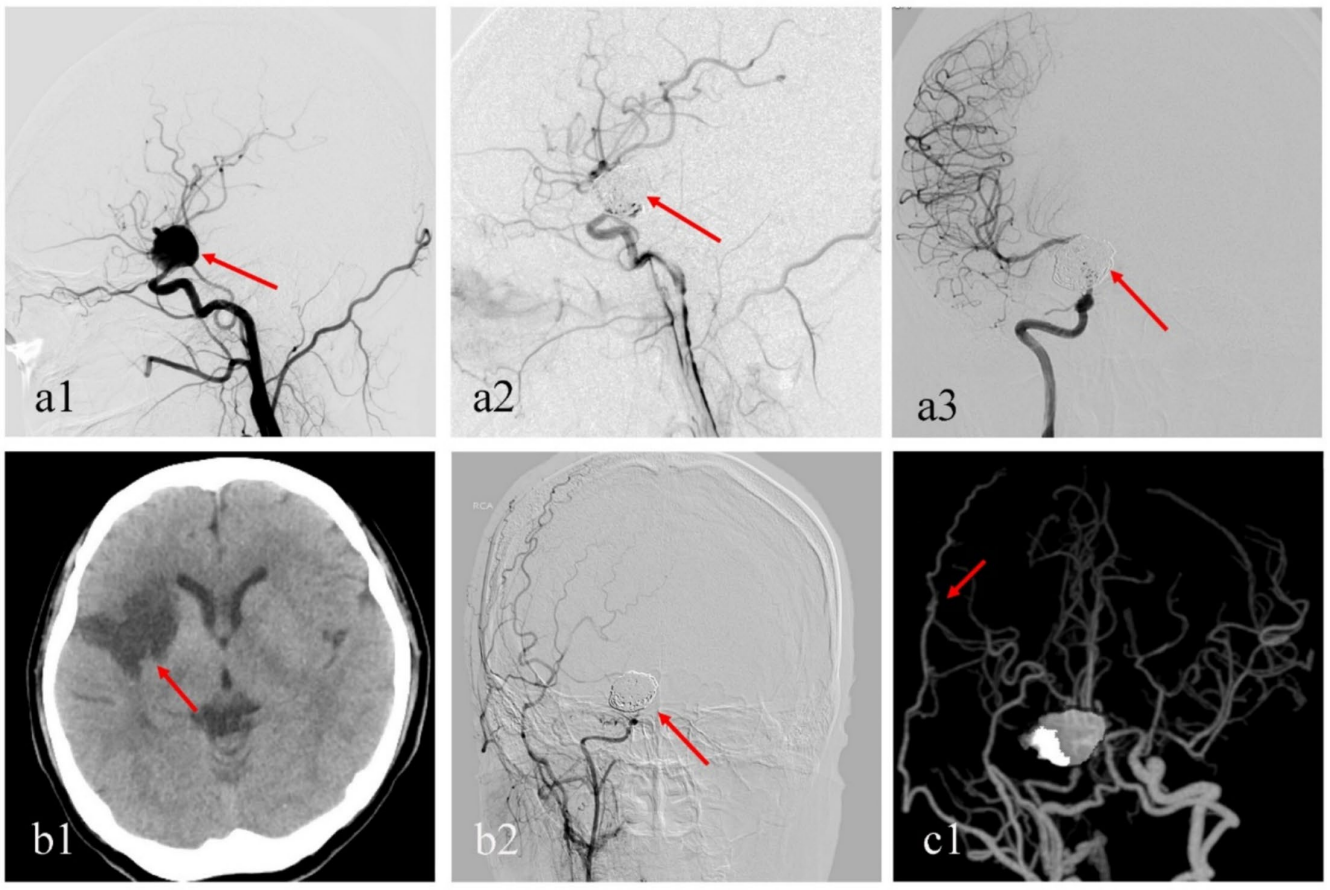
A female patient with a giant aneurysm at the distal end of the right internal carotid artery, approximately 25 mm in size (a1). She underwent a PED (3.0*35 PED Classic) combined with coiling, and immediate postoperative angiography showed aneurysm occlusion (a2). At the 3-month follow-up, the aneurysm was complete occlusion, but there was a suspicion of parent stenosis (a3). Six months post-procedure, the dual antiplatelet therapy switching monotherapy. Ten months post-procedure, she experienced sudden headaches accompanied by projectile vomiting, facial asymmetry, and right-sided hemiplegia, with right cerebral infraction by CT (b1). After rehabilitation, her neurologic function improved (modified Rankin Scale grade 2), but she continues to experience intermittent occurrences of transient ischemic attacks. Follow-up cerebral angiography showed a narrowing at the distal end of the internal carotid artery and the middle cerebral artery (b2). She subsequently underwent extracranial-intracranial bypass surgery and has now fully recovered (c1).
